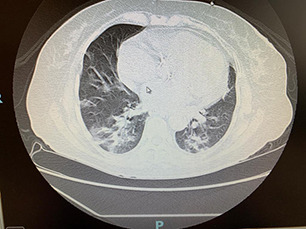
Non- contrast thin-section CT Chest in axial plane, taken at the level of middle and lower lobes, showing the combination of bilateral, asymmetrical, subpleural ground glass pattern, interstitial thickening and consolidation. The involvement is bilateral, and subpleural, with most lesions located in anatomical regions which would be hidden by the mediastinal structures and cardiac silhouette in a frontally projected chest radiograph. (Image courtesy: Dr Imran Sharief, MD, FCCP)
